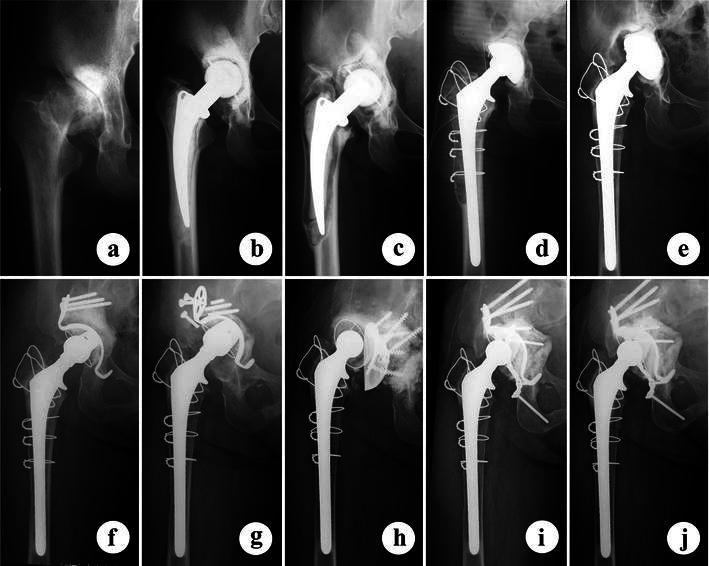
Serial anteroposterior radiographs of the patient who underwent four revision surgeries of the right hip joint. a Radiograph at age 39, showing dysplastic hip joint osteoarthritis; b after primary total hip arthroplasty (THA) at 40 years; c 11 years after the primary THA, showing loosening of both femoral stem and acetabular cup; d immediately after the first revision THA at age 51; e 10 years after the first revised surgery, showing acetabular cup loosening; f after the second revision surgery. The acetabular component was reconstructed with Kerboull reinforcement plate; g 3 years after the second revision surgery, showing breakage of the reinforcement plate and resorption of the graft bone; h after the third revision surgery using Müller ring with impaction bone grafting; i 3 years after the final revision at age of 72; j 7 years after the final revision surgery with stabilization of the two acetabular columns. Note healing of the pelvic discontinuity lesion
